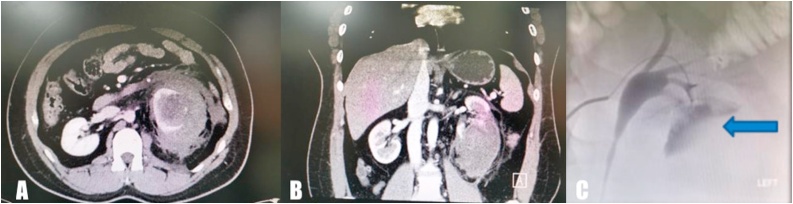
A, B: a computed tomography (CT) scan which revealed a 9.7 × 9 × 11 cm left perirenal hematoma mainly occupying the lower pole of the left kidney. C: angiogram during emergency embolization which revealed an area of extravasation.
